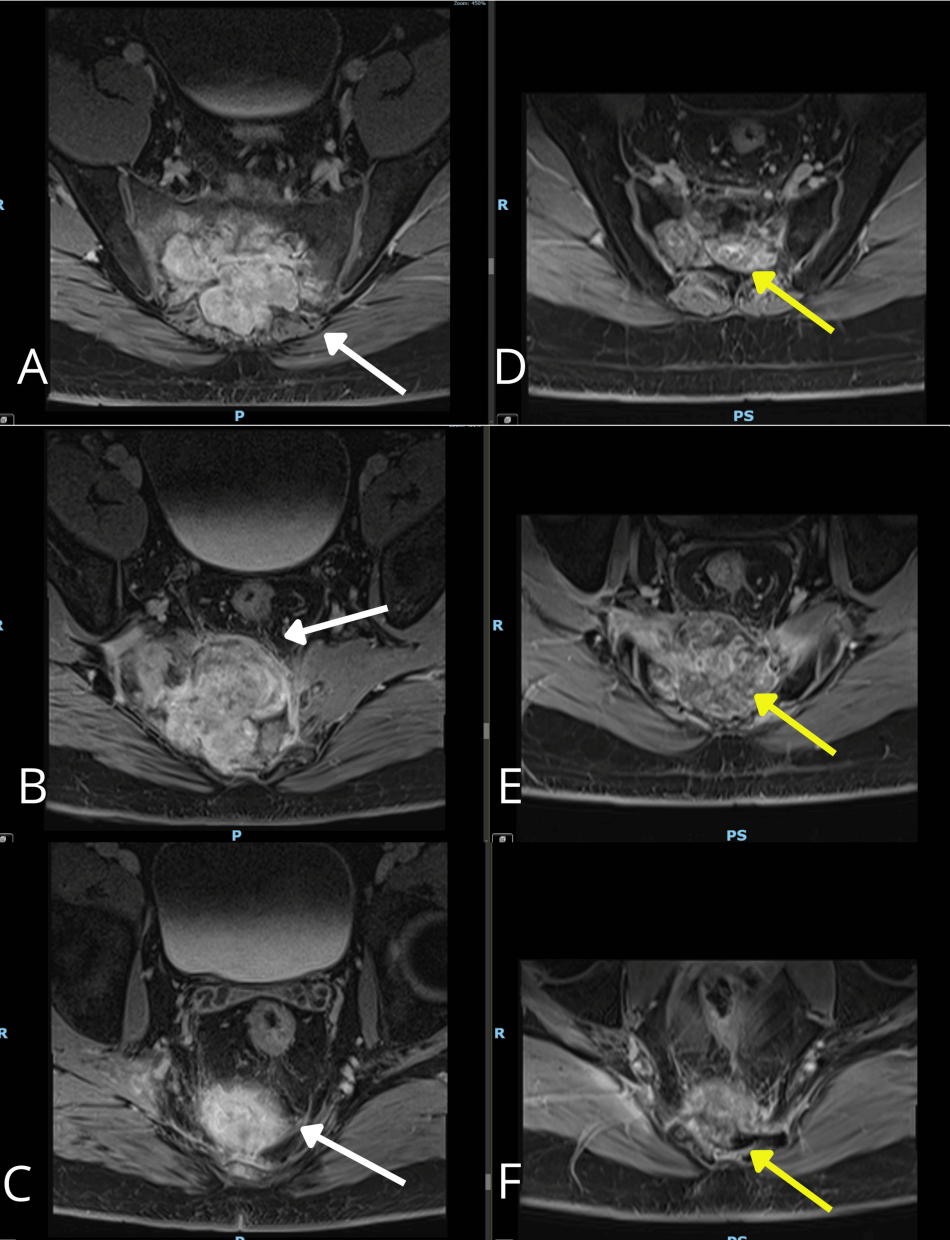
Enhanced-contrast MRI images of the sacral tumor. (A-C) Before the treatment in 2023. (D-F) After robotic radiosurgery in 2024. The white arrow in A, B, and C indicates the sacral mass before treatment. The yellow arrow highlights the reduction in the size of the sacral mass and the increasing separation from the rectum after treatment.
